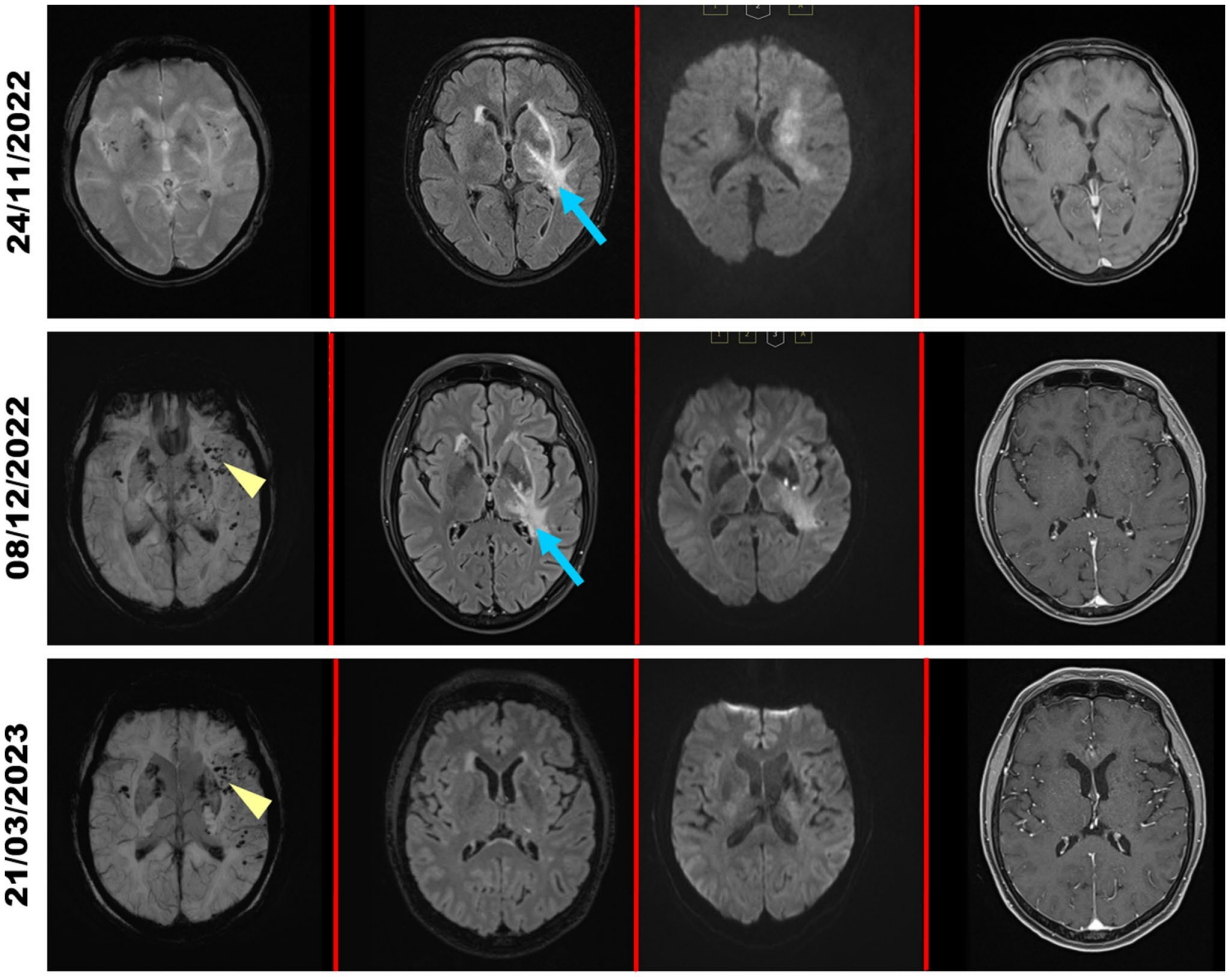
MRI scans during the clinical course. MRI scans of the patient at admission (24/11/2022; upper row), during corticosteroid treatment (08/12/2022; middle row) and in clinical follow-up in March 2023 (21/03/2023; lower row). Images were acquired (from left to right) in the SWI, FLAIR, DWI (*b* value = 1,000 s/mm^2^) and contrast-enhanced T1 sequence. At admission, the left frontotemporal region shows a pronounced frontotemporal edema (blue arrows) with significant reduction under corticosteroid treatment and complete regression in the clinical follow-up 03/2023. The SWI sequence shows microhemorrhages (yellow arrowheads) as a sign of newly diagnosed CAA-ri.
